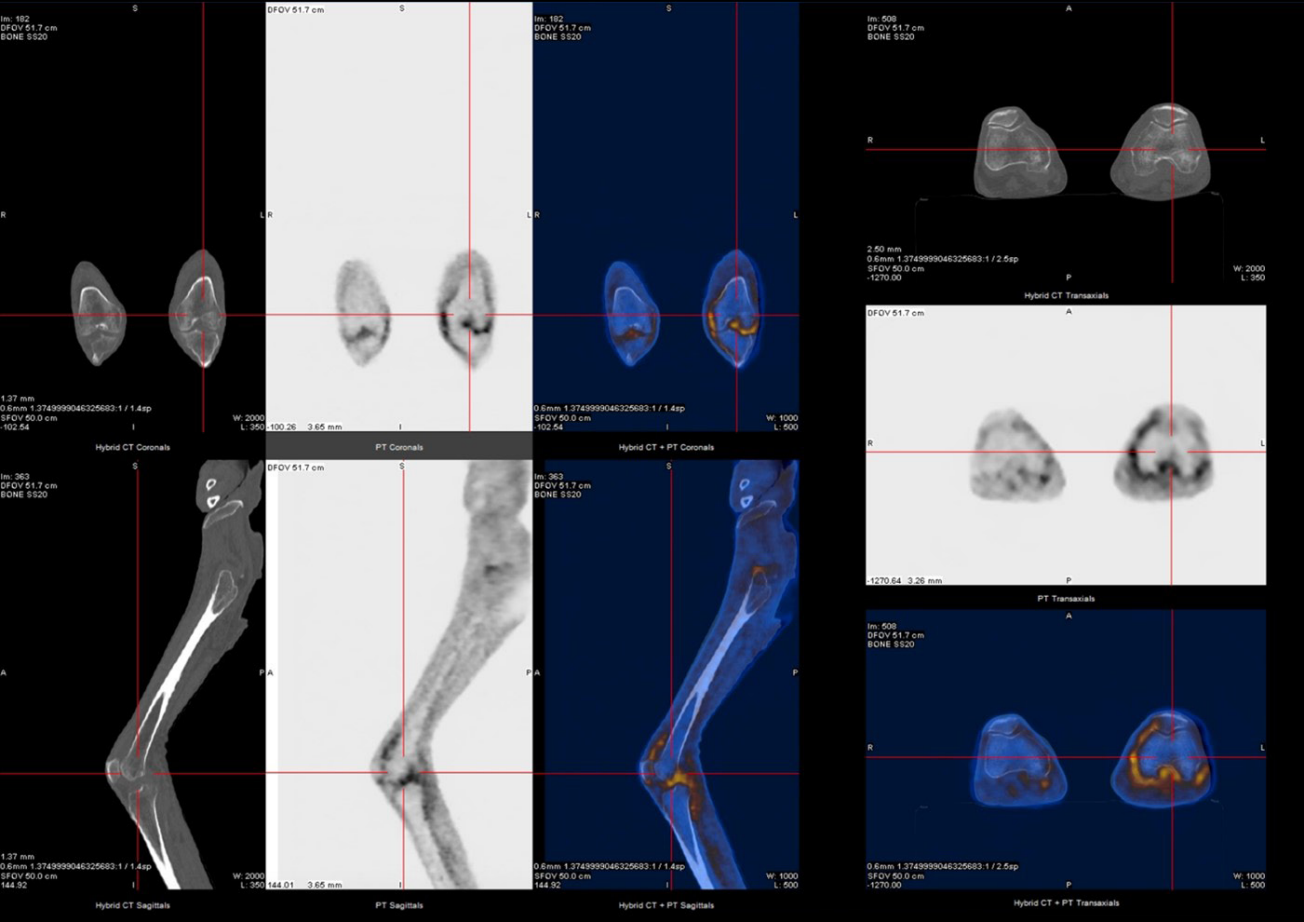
18F-FDG and PET-CT study reveals slightly increased uptake of FDG in the knee joints (more in the left knee joint, with apparent extension to the subquadricipital synovial bursa region). PET-CT, pistron emission tomography-computed tomography*.
